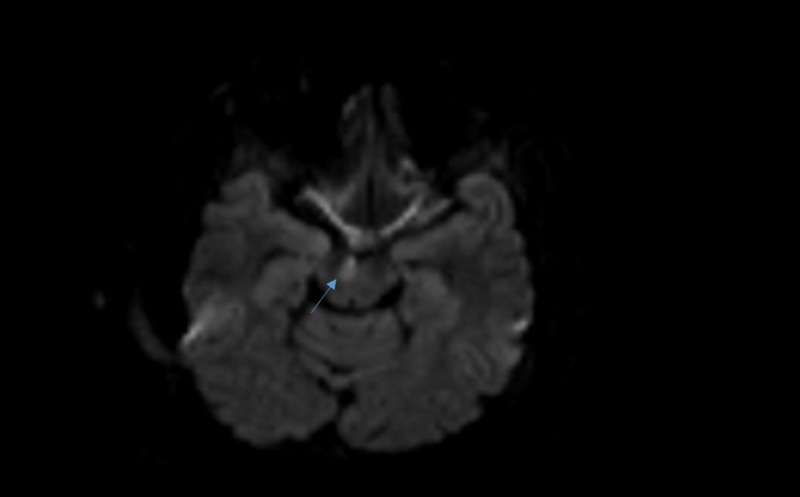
Magnetic resonance imaging showing evidence of a stroke in the right periaqueductal gray
